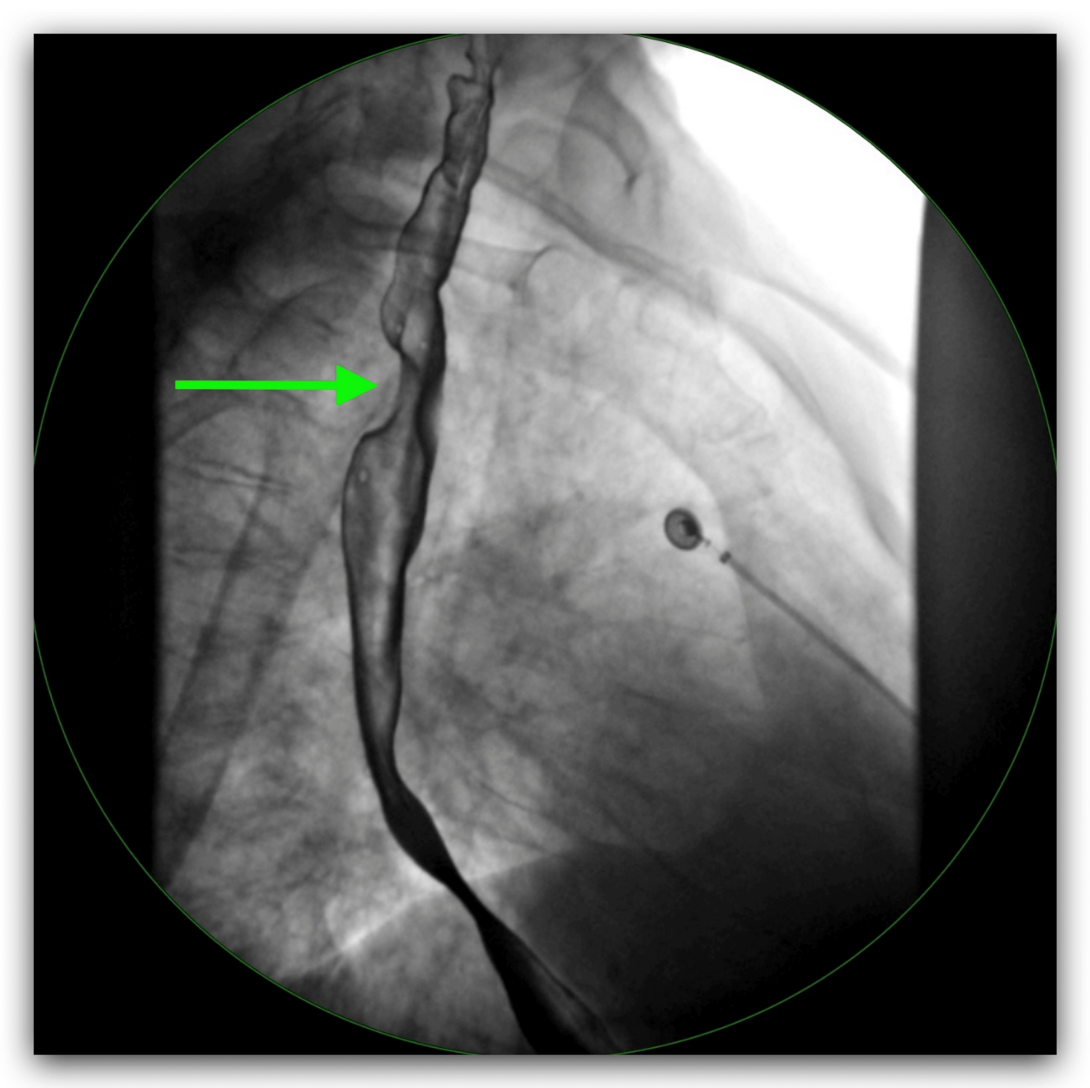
Upper gastrointestinal series X-ray with esophagram showed a small hiatal hernia, gastroesophageal reflux, and the green arrow indicates extrinsic compression of the lumen of the esophagus and the level of the subclavian artery
